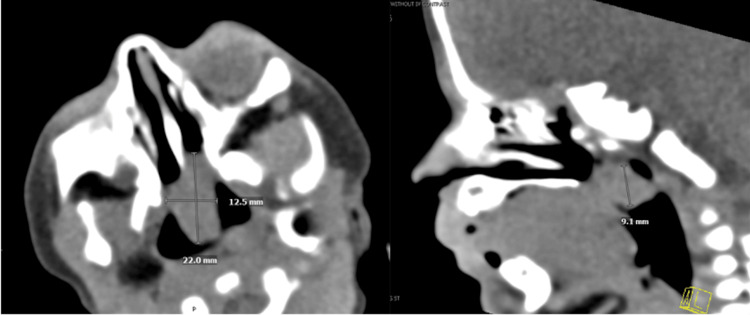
CT facial bone done on day of life 12. Depicts the dimensions of the mass. Nonspecific 2.2 cm polypoid soft tissue inseparable from the soft palate with extension into the nasopharynx, oropharynx, and bilateral coanae. No associated bone destruction.
